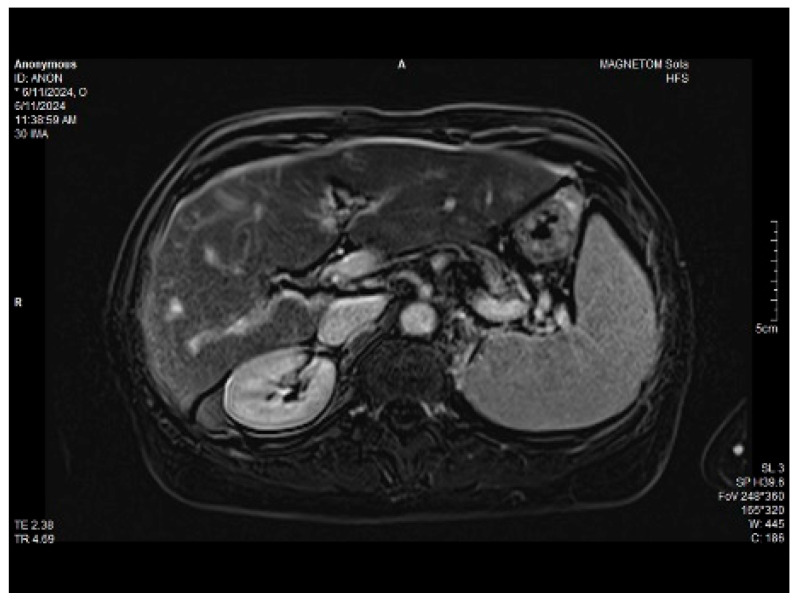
Small veno-portal shunts visible at the periphery of both hepatic lobes: MRI aspects.
